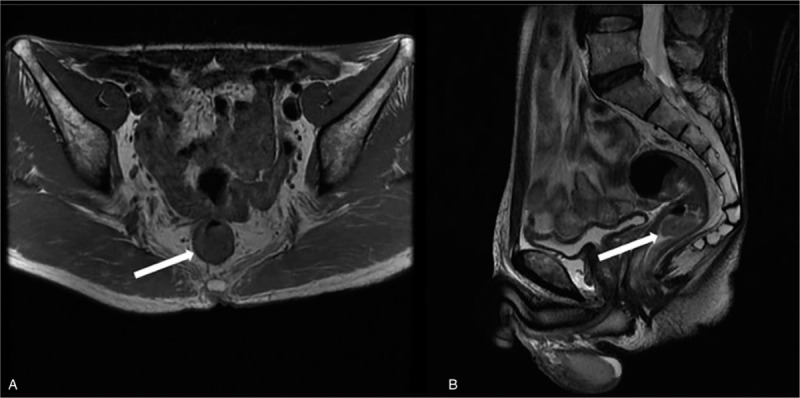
The manifestation of magnetic resonance imaging. (A) T1 manifestation in cross section view. The tumor size was 23 mm × 10 mm × 10 mm (with white arrow). (B) T2 manifestation in sagittal view. The distance from distal edge of tumor to anal edge was around 55 mm (tumor with white arrow).
